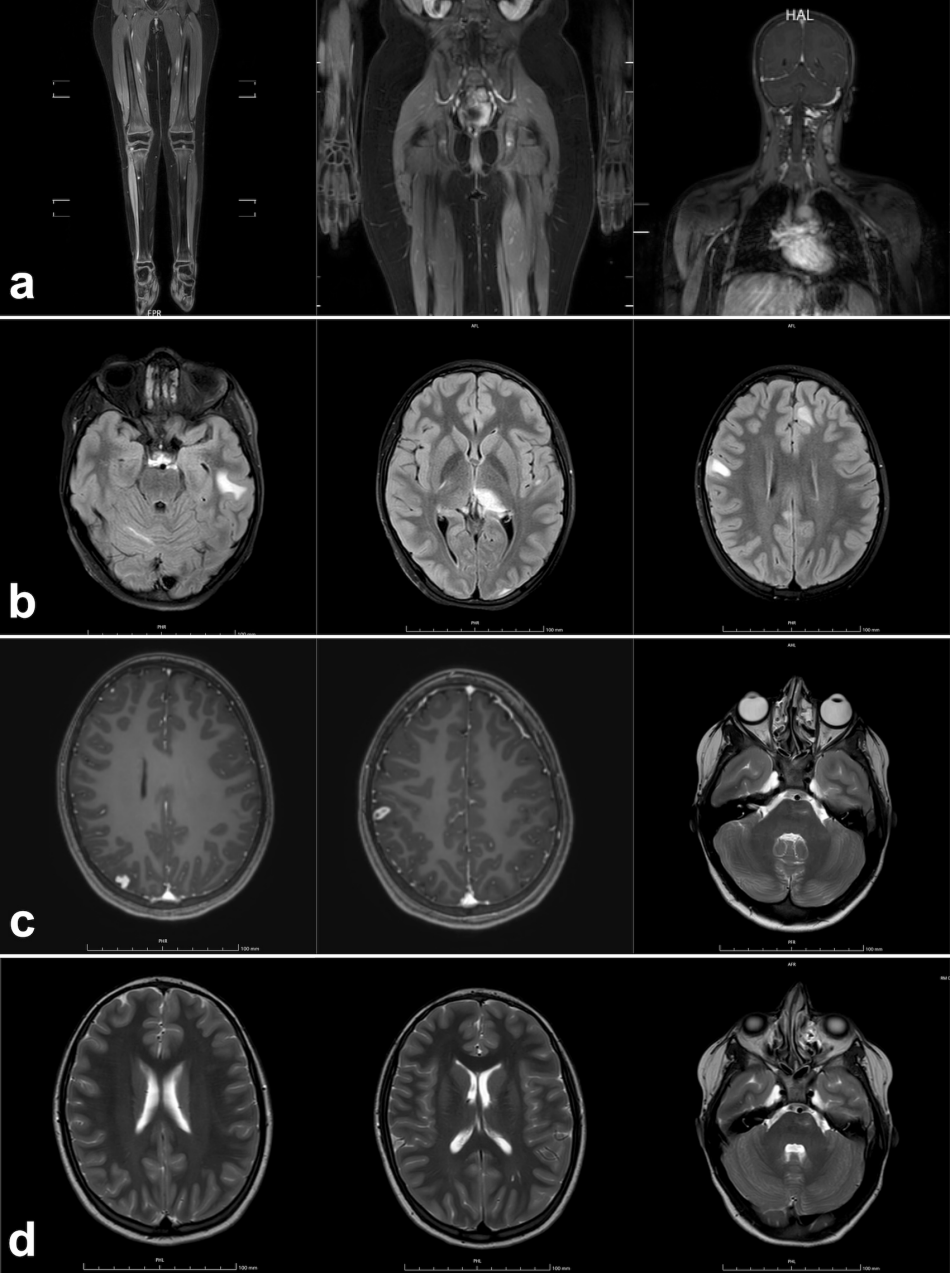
Detail of the initial whole-body MRI of a 11 years-old female patient with Mycobacterium avium complex (MAC) infection and sarcoid-like lesions, showing multiple bone lesions in the tibial diaphyses and epiphyses, left femoral diaphysis, ischial punctate lesions, and left cervical lymph node involvement (a). Brain MRI performed during disease exacerbation, 9 months after the initial whole-body MRI, revealed multiple areas of signal alteration in the right cerebellar hemisphere, left temporal and frontal subcortical white matter, splenium, and left thalamus (b). Overall reduction of the previously noted areas of signal alteration, apart from a small new hyperintense lesion in the right parietal region (c), observed 4 months after the initiation of triple antimycobacterial therapy. Further reduction of the known alterations was noted, with stable size of the left pontine lesion (d), assessed 3 months later.
